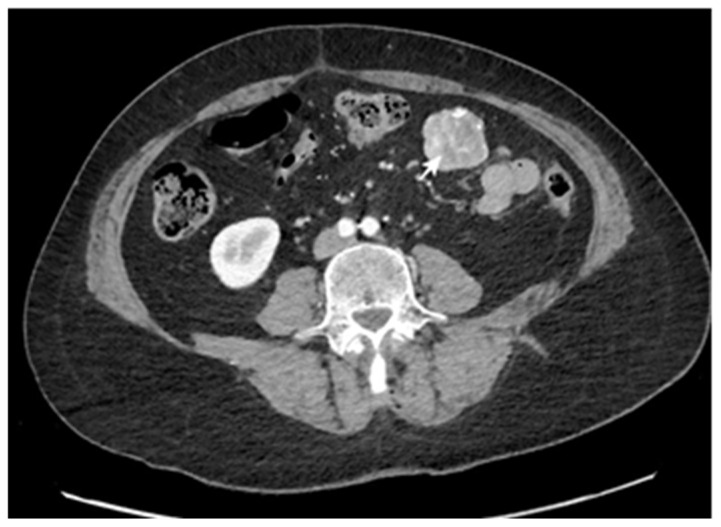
CT scan post-contrast with heterogeneous uptake and central necrosis, vascular peripheral enhancement, and regular contour, with no loco-regional extension. The pathology exam revealed an intestinal stromal tumor.
